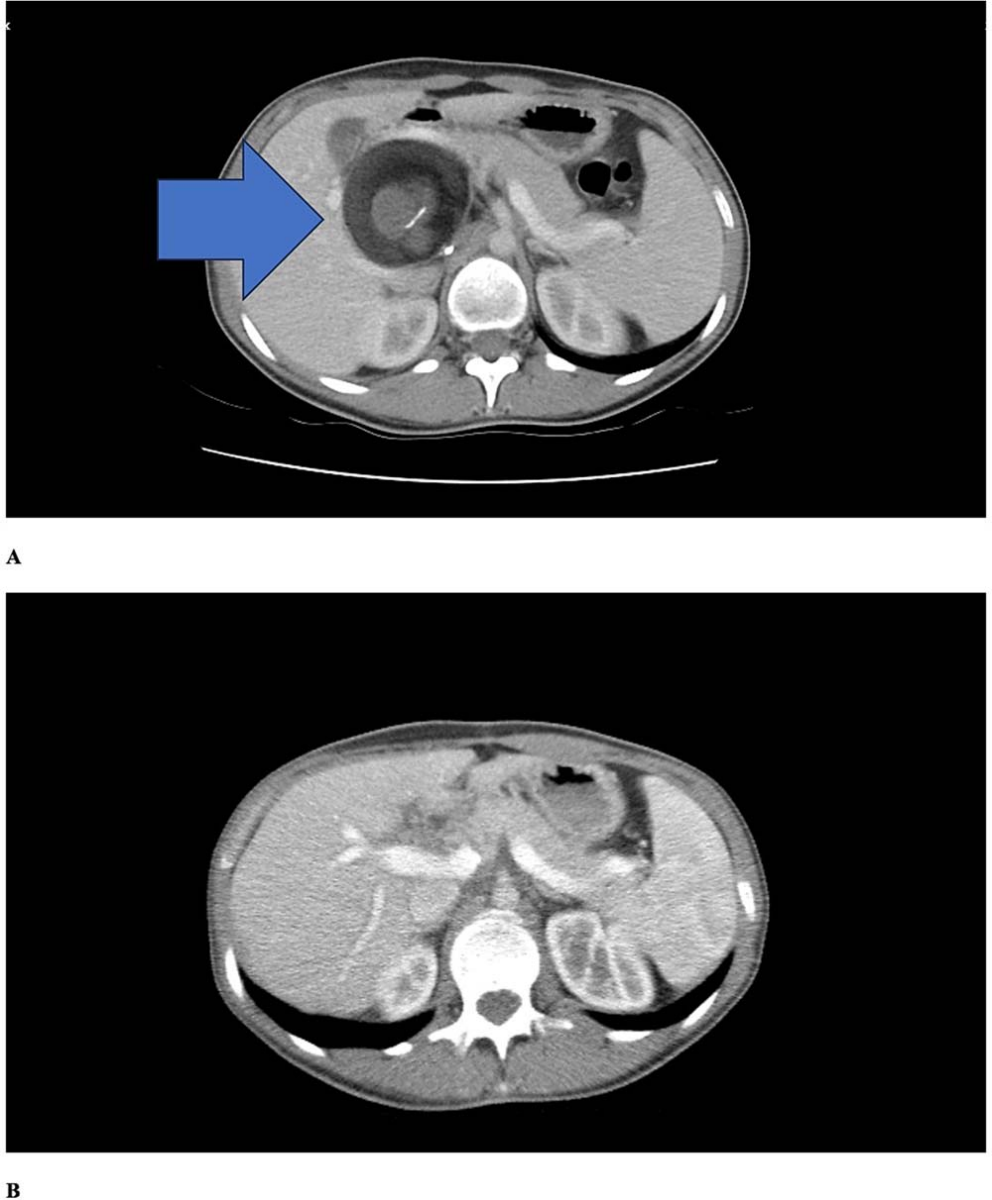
Contrast-enhanced axial CT scans. (a) Preoperative imaging showing a well-defined cystic lesion in the hepatoduodenal ligament region with heterogeneous attenuation including areas of fat density (negative Hounsfield units) and fluid components. (b) Six-month postoperative follow-up demonstrating complete resolution of the lesion with no evidence of recurrence. Periportal millimetric metallic densities representing surgical clips are visible.
